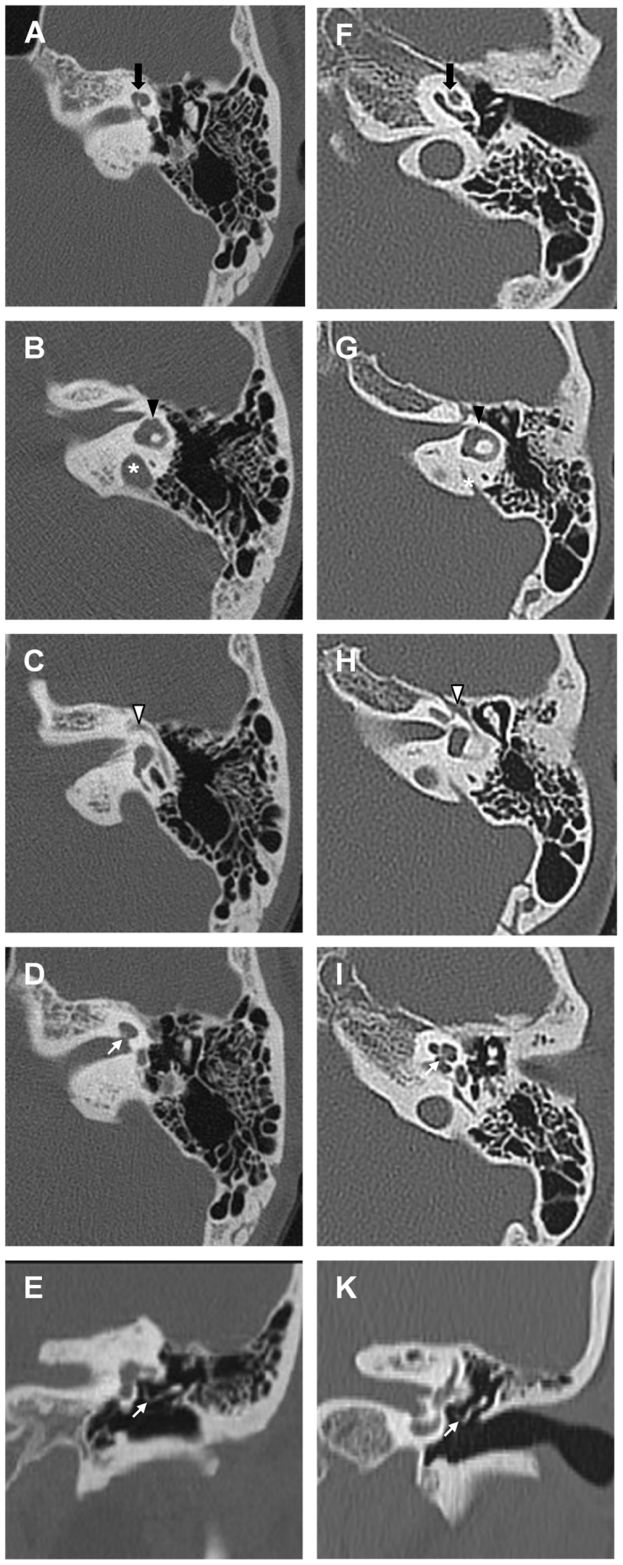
The findings of temporal bone CT in patient 7 and normal control. (A–E) These images are temporal bone CT in patient 7. (A) Cochlear hypoplasia type III with less than two turns is indicated by a black arrow. (B) The vestibular aqueduct (white asterisk) was enlarged and seen in a circular shape in the axial view. The lateral semicircular canal was slightly hypoplastic and the vestibular was dilated (black arrowhead). (C) The facial nerve ran inferior to the hypoplastic cochlea and displayed an obtuse angle between the labyrinthine and tympanic segments (white arrowhead). (D) The modiolus (white arrow) was present but defective and hypoplastic. (E) The ossicular chain (white arrow) seen in the coronal view was positioned in a different angle compared to the normal control. (F–K) These images are temporal bone CT in normal control.
